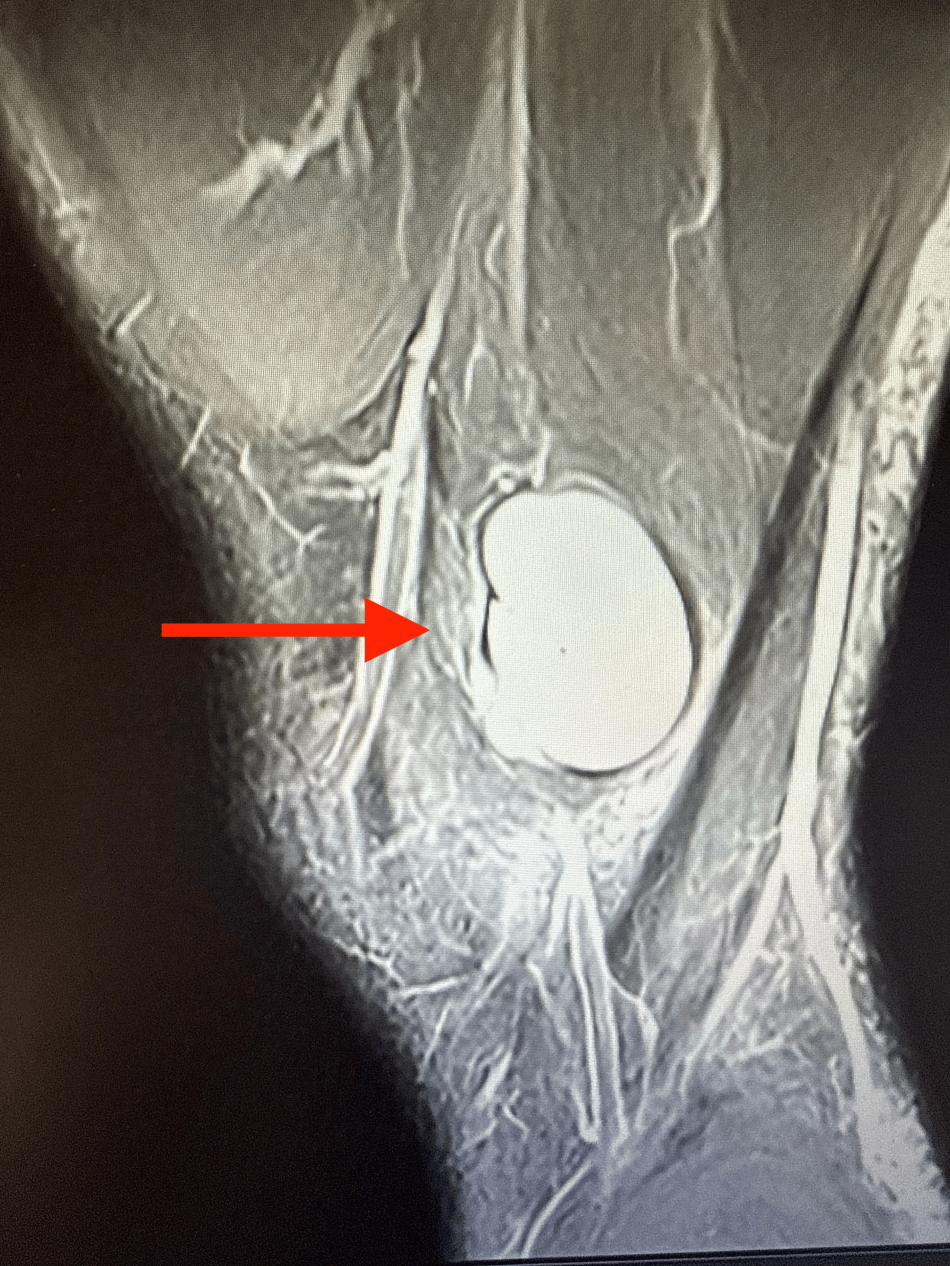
MRI of the knee showing hyperdensity with sharp demarcating edges suggesting fluid collection in the distal thigh (arrow).
